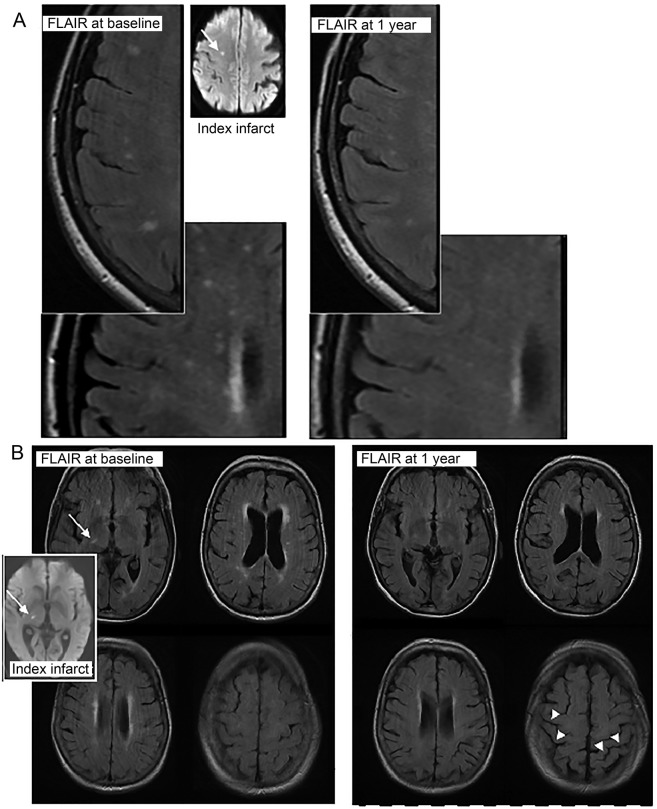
Examples of WMH reduction in 2 participants MRIs from patients (A, top and B, bottom) who showed definite visible reduction in WMH on MRIs between presentation with minor stroke (left, baseline; inset, the acute index infarct [arrow] on MRI diffusion tensor imaging) and 1 year (right). Note also the increase in visibility of sulci at 1 year (arrowheads, B, bottom right), indicating a reduction in brain volume accompanying the reduction in WMH volume. FLAIR = fluid-attenuated inversion recovery; WMH = white matter hyperintensities.
